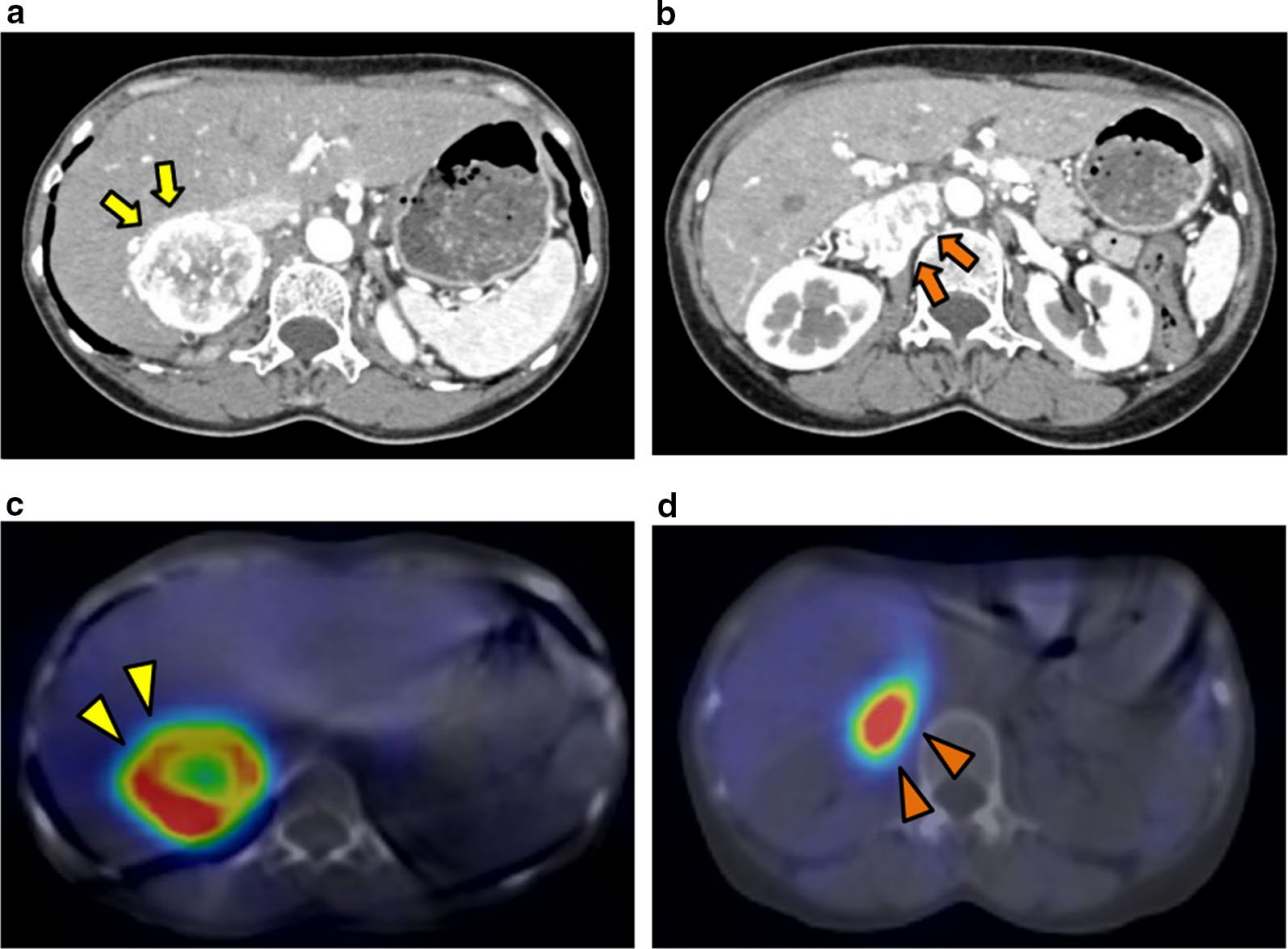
Abdominal computed tomography at the first visit shows the right adrenal gland tumor (a, yellow arrows) and the lymph node metastasis (b, orange arrows) on the hilum of the right kidney. ^123^I-metaiodobenzylguanidine (MIBG) scintigraphy shows MIBG in the adrenal tumor (c, yellow arrowheads) and lymph node metastasis (d, orange arrowheads)
